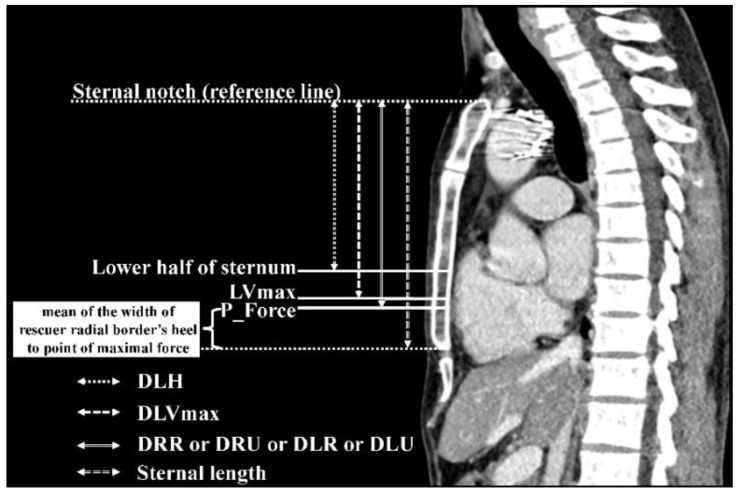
Sagittal view of chest computed tomography. P_Force indicated the point of the maximal chest compression force of the rescuer’s hand heel which placed the lowest palmar margin of the rescuer’s hand at the xiphisternal junction (lower end of the sternum). Abbreviations: DLH, distance from the sternal notch to the lower half of sternum; DLVmax, distance from the sternal notch to the point of maximal left ventricular diameter on the sternum; DLR, distance from the sternal notch to the point of maximal force of the rescuer’s left hand with the radial border located at the xiphisternal junction; DLU, distance from the sternal notch to the point of maximal force of the rescuer’s left hand with the ulnar border located at the xiphisternal junction; DRR, distance from the sternal notch to the point of maximal force of the rescuer’s right hand with the radial border located at the xiphisternal junction; DRU, distance from the sternal notch to the point of maximal force of the rescuer’s right hand with the ulnar border located at the xiphisternal junction; LVmax, The point of maximal left ventricular diameter on the sternum.
